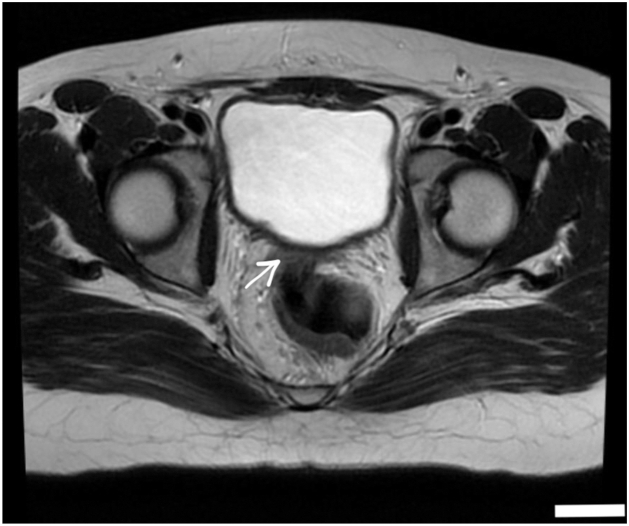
Axial pelvic MRI image showing an absent uterus (arrow) located posterior to the bladder. Ovaries are not visualized. The urinary bladder is distended with a normal wall and adjacent musculature. No pelvic mass was detected.
